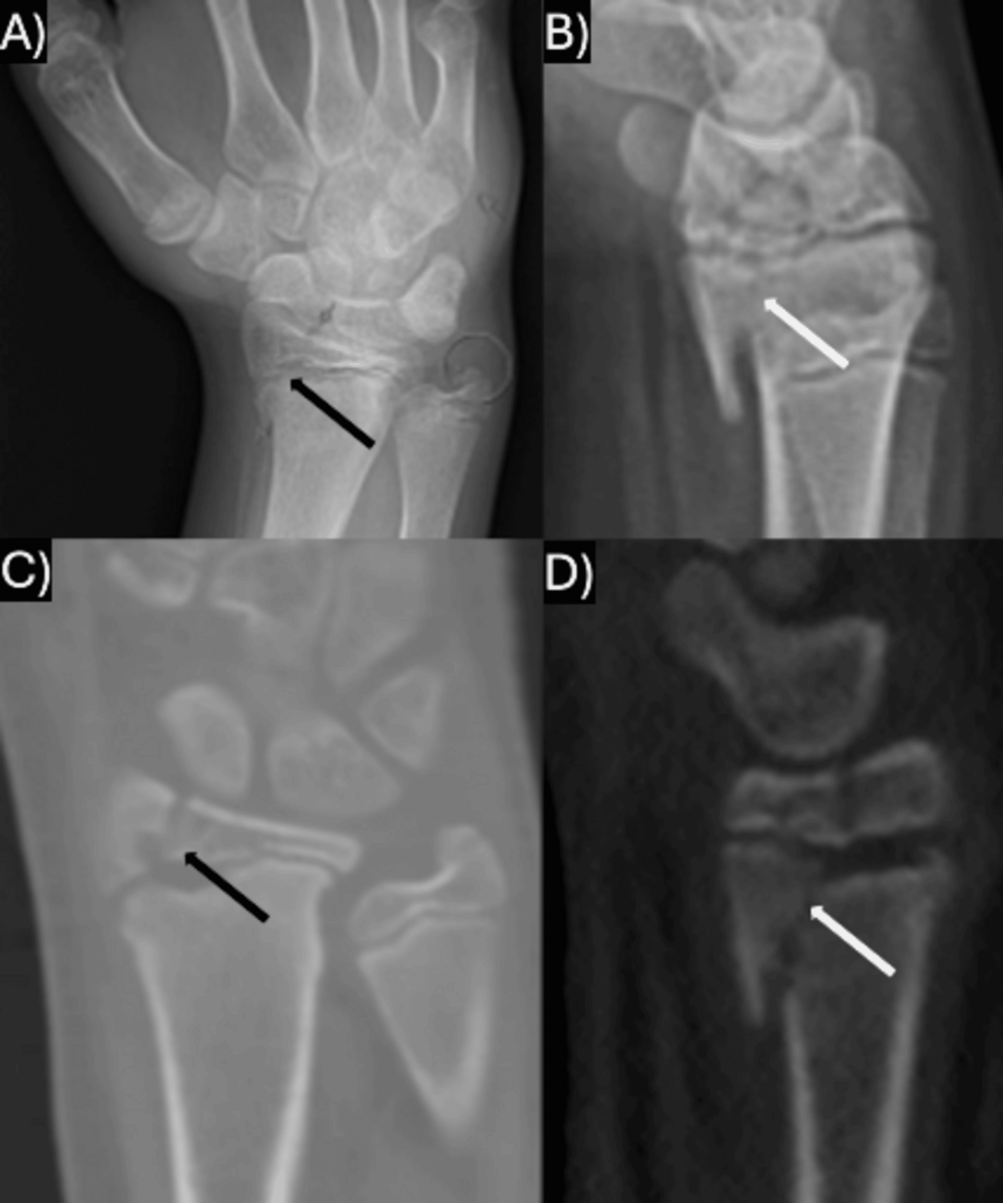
Imaging from case II: this was the only injury with a radial-based fracture as well as an SH-IV fracture line on the lateral radiographs. A) Case II's AP radiographs; B) Case II's lateral radiographs; C) Case II's coronal CT imaging; D) Case II's sagittal CT imaging. In all panels, the black arrows demonstrate the AP radiograph/coronal CT fracture line, while the white arrows demonstrate the lateral radiograph/sagittal CT fracture line. SH: Salter-Harris; AP: anterior-posterior
